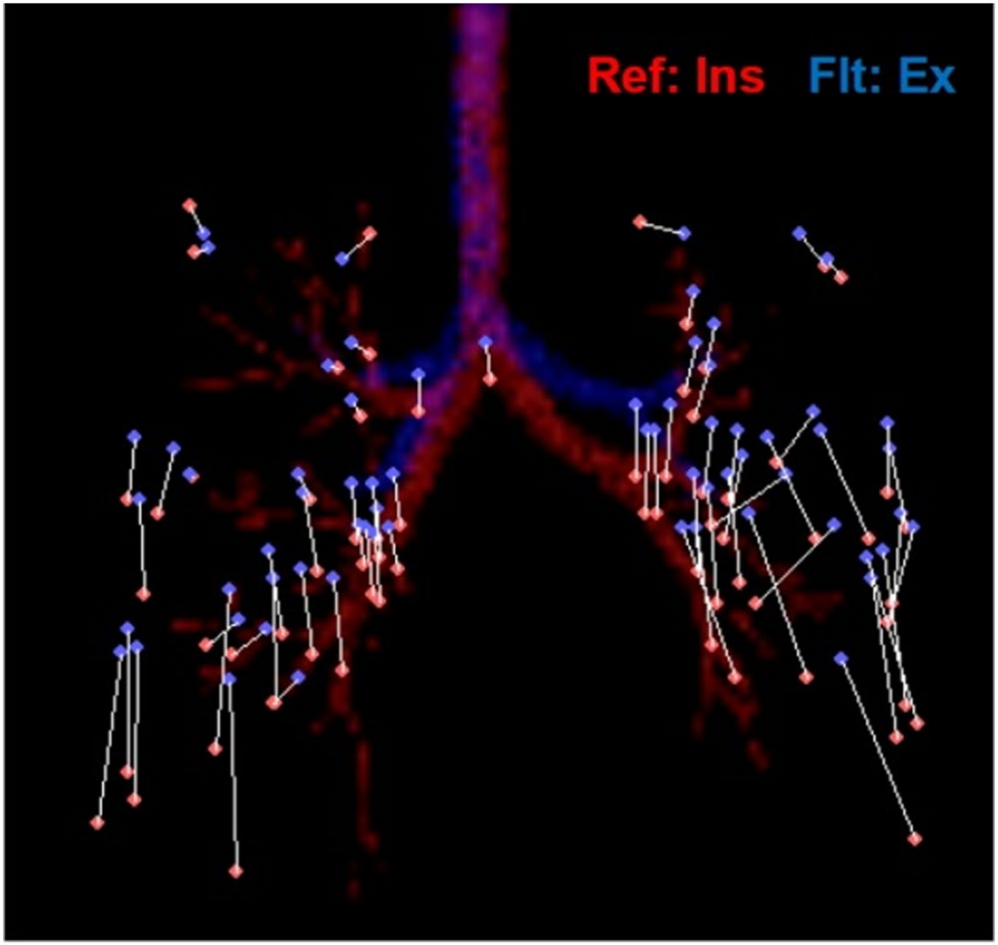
A representative map of landmarks of the bronchial tree and pulmonary vessels. In this patient, a total of 67 landmarks (33 in right lung, 33 in left lung, and on at carina) were placed in a side-by-side display of 1-mm-thick inspiratory and expiratory CT images. The landmarks in the inspiratory (red dots) and expiratory CT (blue dots) are paired to represent the same location.
